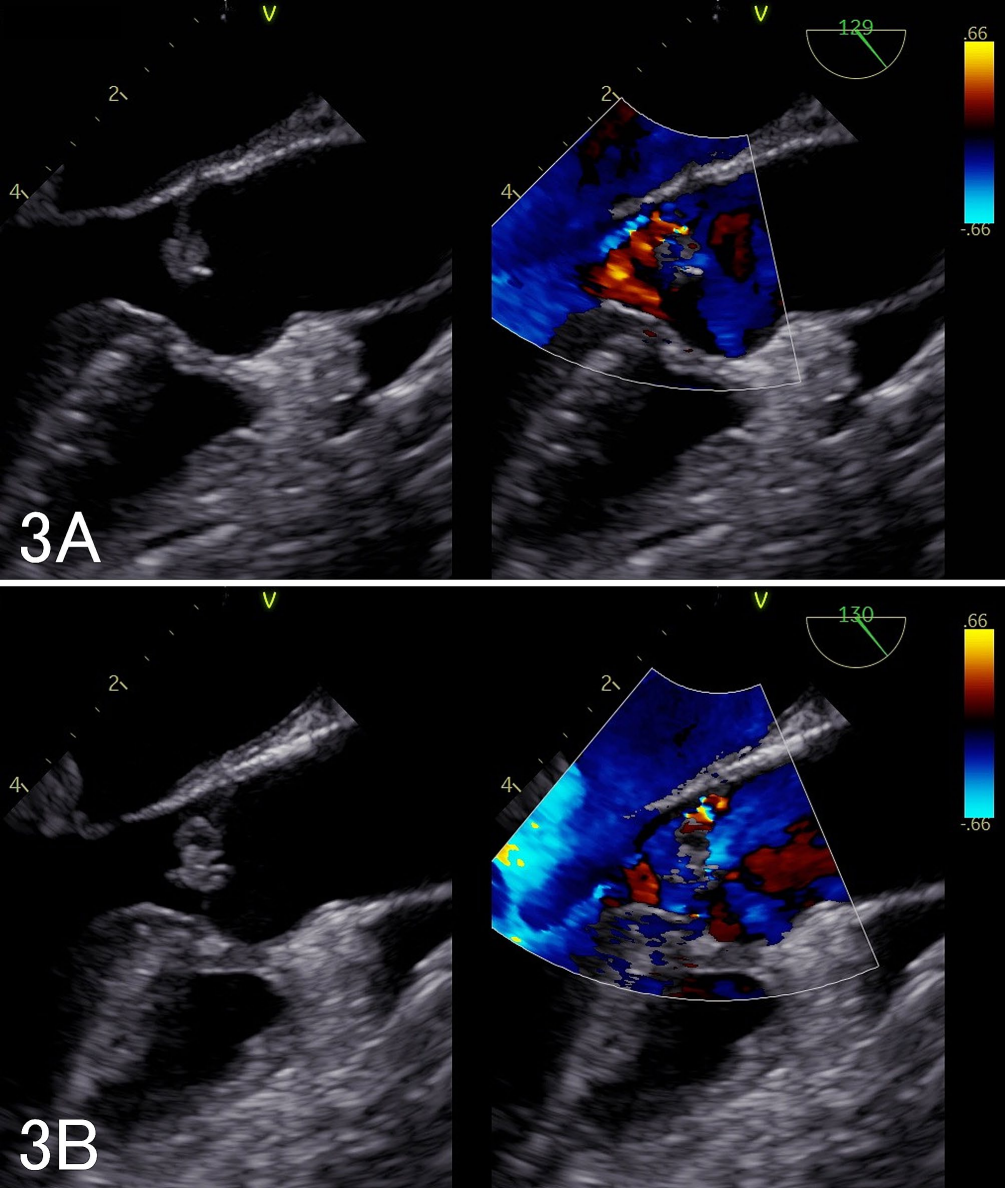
Comparison of aortic valve long-axis images. A: Post-ICU admission transesophageal echocardiography (TEE): verrucae adhered to the aortic valve and aortic regurgitation were observed. B: Preoperative TEE showing enlarged verrucae and worsened aortic regurgitation
